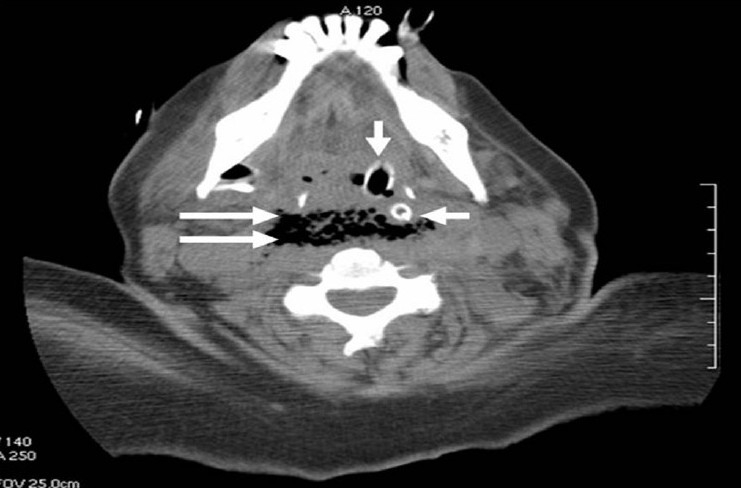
An axial computed tomogram (non-enhanced) reveals free air and air bubbles within the dangerous space (two horizontal arrows), ET tube (vertical arrow) and NG tube (one horizontal arrow)
